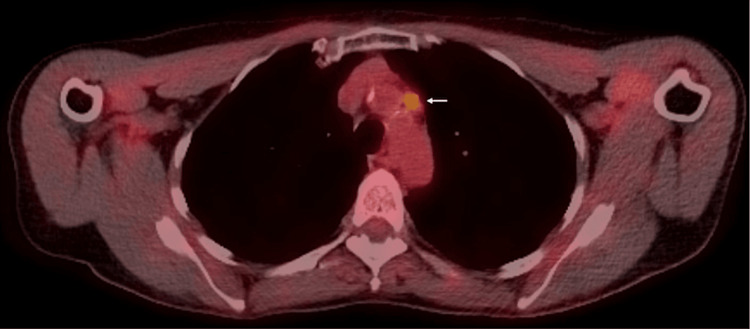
PET/CT completed in February 2022 demonstrating an increased FDG uptake in mediastinal mass (arrow)
